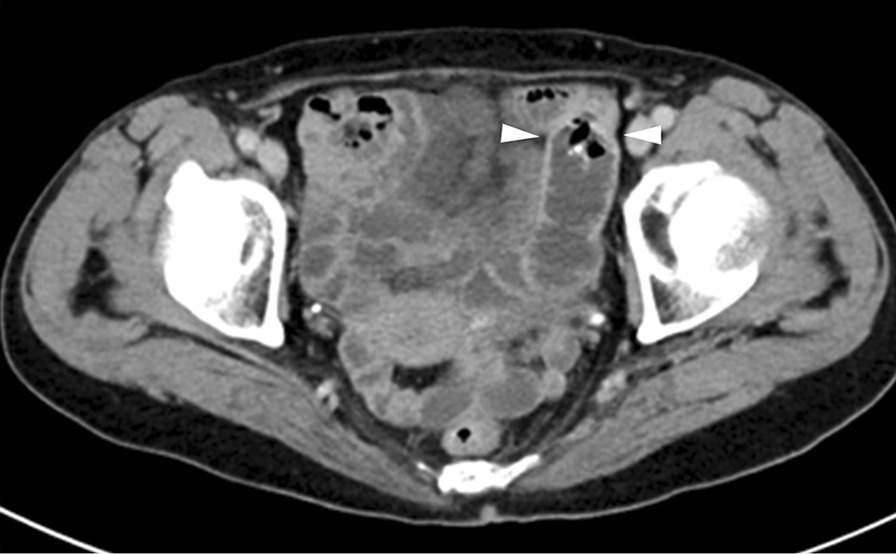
Abdominal CT showed the patency capsule stagnated in the proximal part of the ileum (arrow head) and mild dilatation of the oral part of the small intestine
